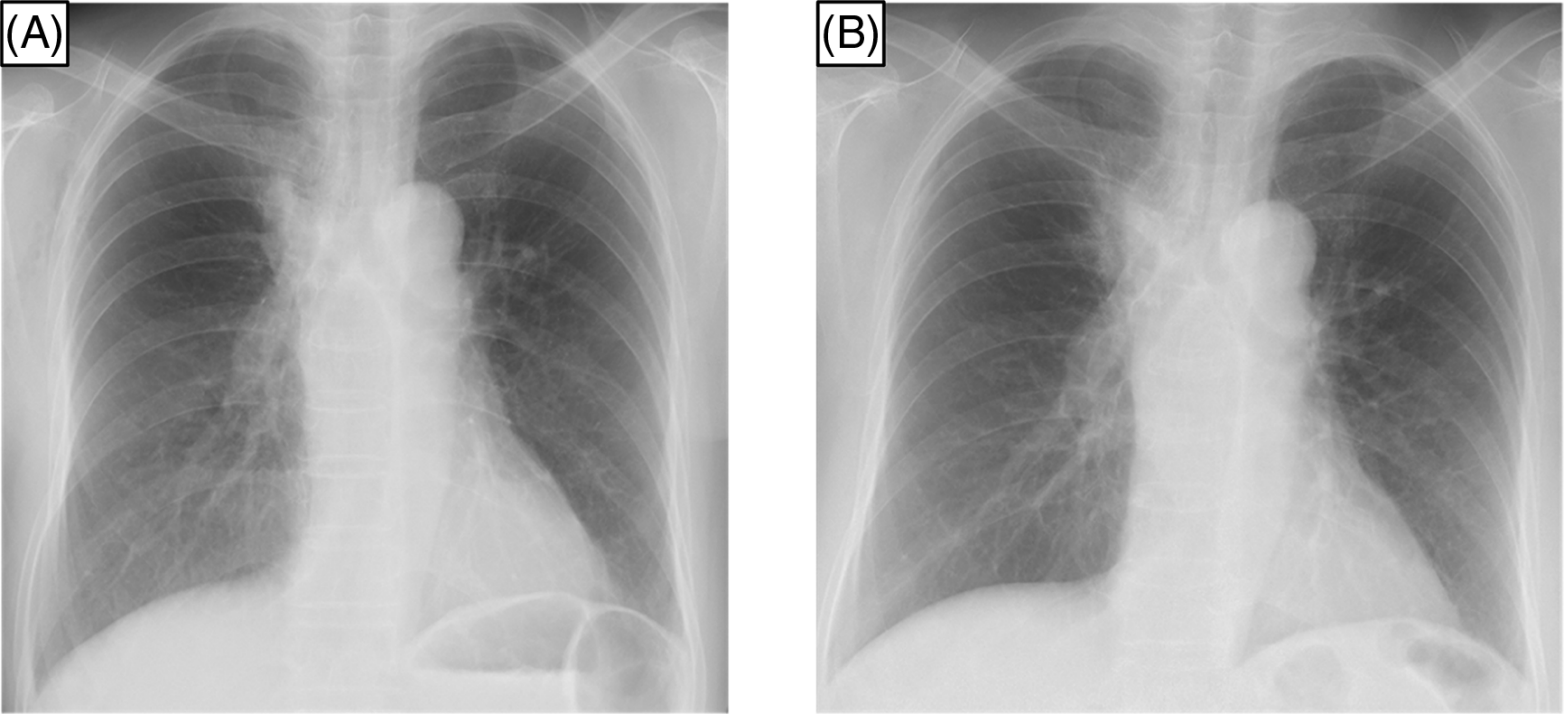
Results of chest radiography imaging. (A) Chest radiograph before starting the sixth treatment, revealing infiltrative shadows in the right upper lung field. (B) Chest radiograph at the time of admission, depicting an increase in the size of the infiltrative shadows.
